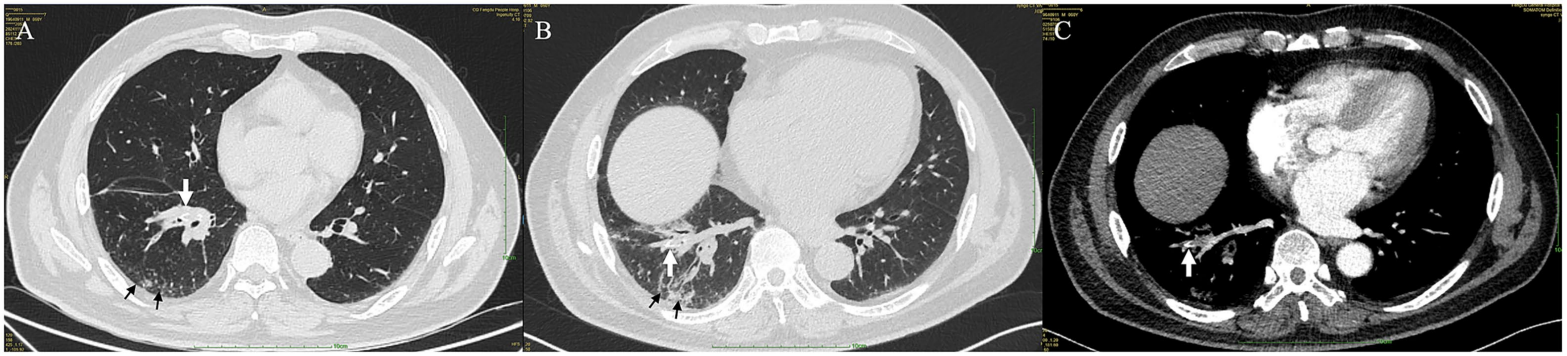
Serial chest computed tomography (CT) imaging of Case 1. (A) Initial axial CT scan (lung window) demonstrated circumferential wall thickening and narrowing of the right lower lobe bronchus (white arrow), with distal patchy ground-glass opacities (black arrow). Note the V-shaped hyperdense opacity (white arrowhead). (B) Follow-up axial CT scan (lung window) 8 months later revealed progression of bronchial wall thickening and luminal narrowing (white arrow), increased peripheral consolidation (black arrow), and distal migration of the V-shaped hyperdense opacity (white arrowhead). (C) Mediastinal-window setting of the follow-up CT scan more clearly visualized the V-shaped hyperdense opacity (white arrowhead), highly suggestive of an aspirated foreign body.
